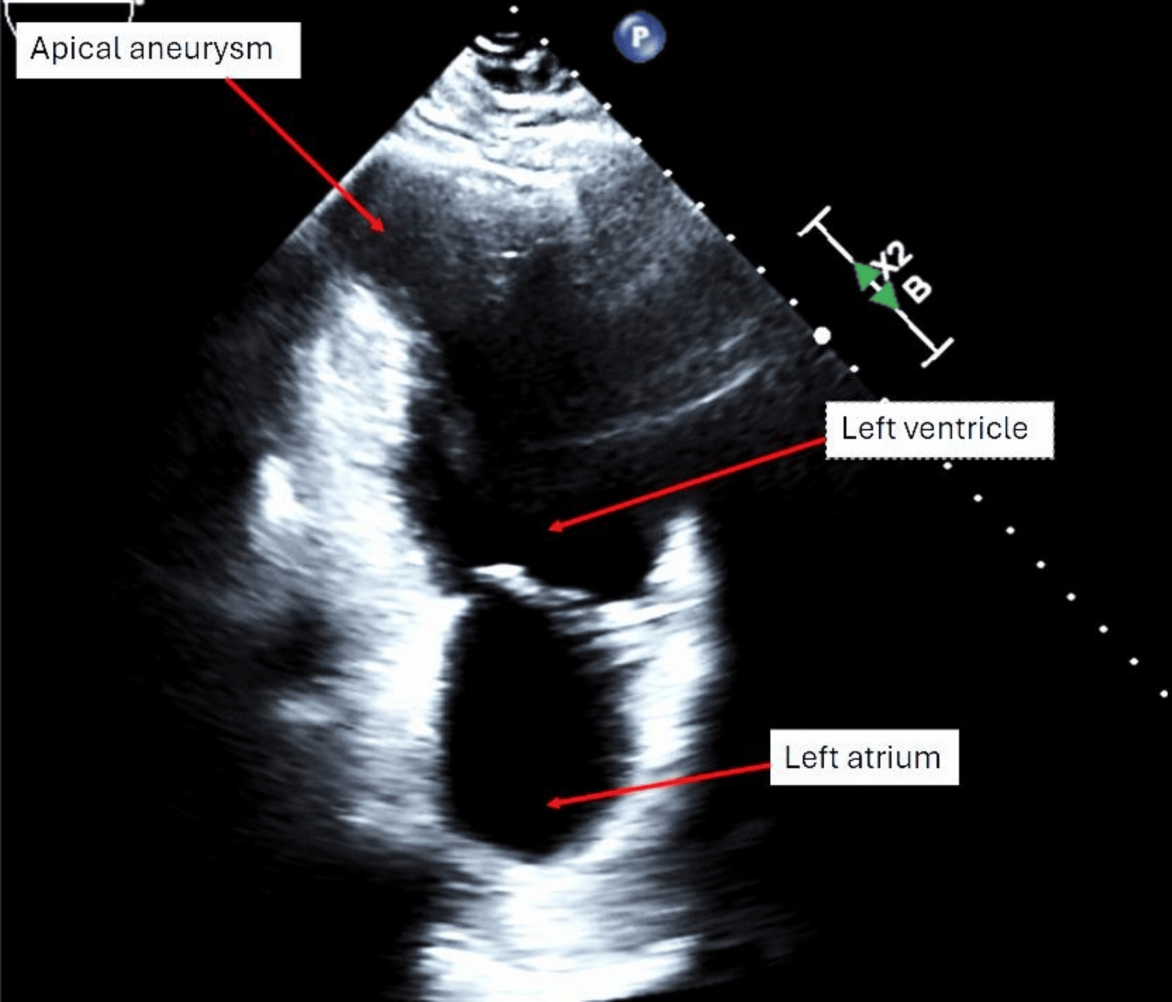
Echocardiogram from June 30 depicting an apical aneurysm.
